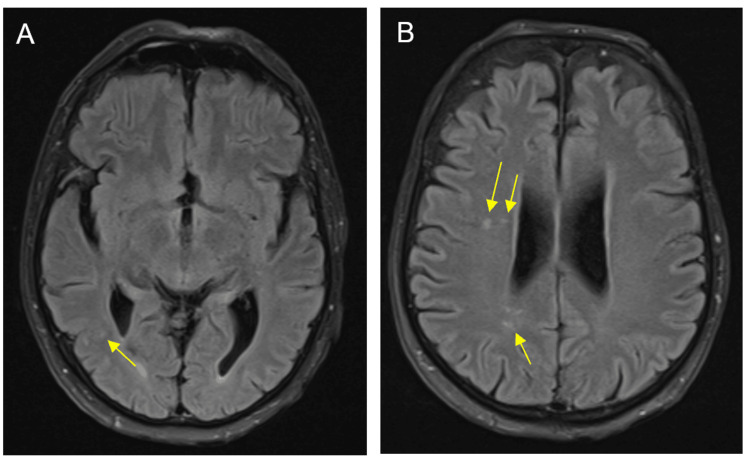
Cranial magnetic resonance Cranial magnetic resonance axial images (A and B) show generalized atrophy and nonspecific foci of hypersignal in the right radial topography and in the right inferior cerebellar hemispherical topography on T2-weighted/FLAIR images (arrows). These later alterations have been previously described in patients with neurosyphilis, however, it cannot be ruled out that these findings could be due to chronic microvascular changes. FLAIR: fluid-attenuated inversion recovery
